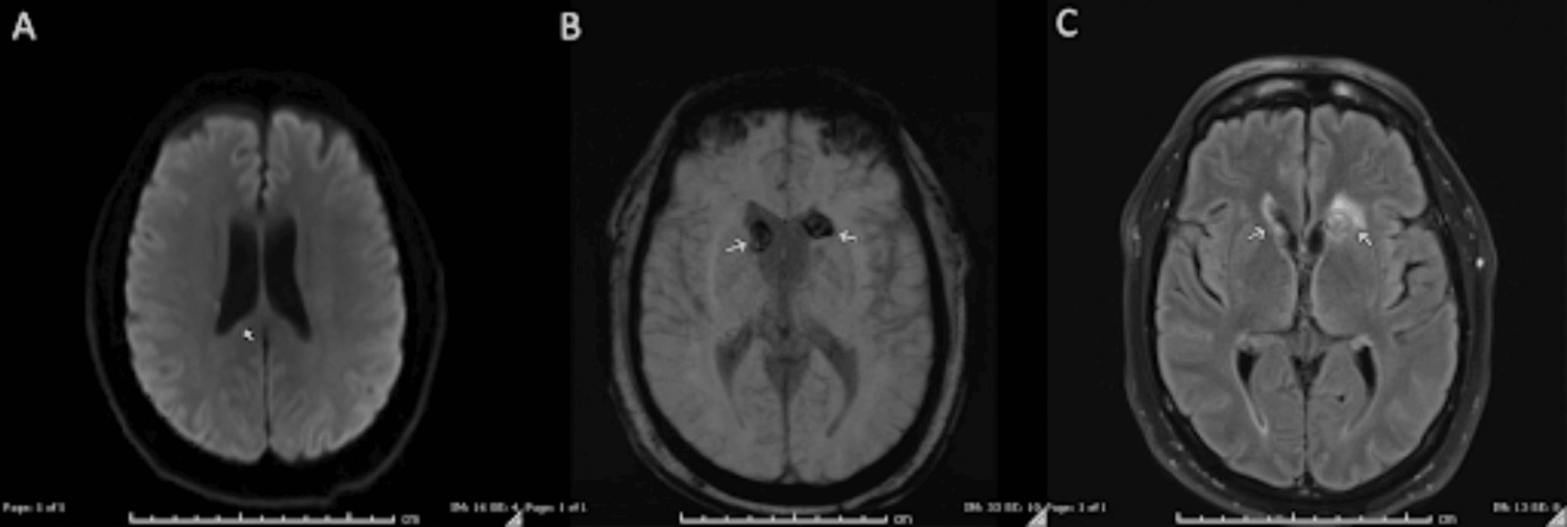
Repeat MRI 99 days after the original MRI (A) DWI: Interval near-complete resolution of the focus of restricted diffusion in the right aspect of the splenium of the corpus callosum. (B) SWI: Interval enlargement of susceptibility artifacts in the caudate heads compatible with hemosiderin deposition/underlying hemorrhage. (C) FLAIR: Decreased abnormal FLAIR signal in the caudate heads. MRI: magnetic resonance imaging; DWI: diffusion-weighted imaging; SWI: susceptibility-weighted imaging; FLAIR: fluid-attenuated inversion recovery
